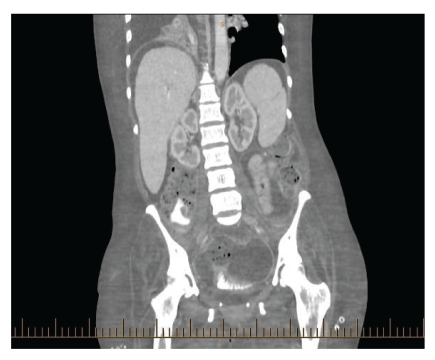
CT scan showing rectosigmoid out-pouching.
